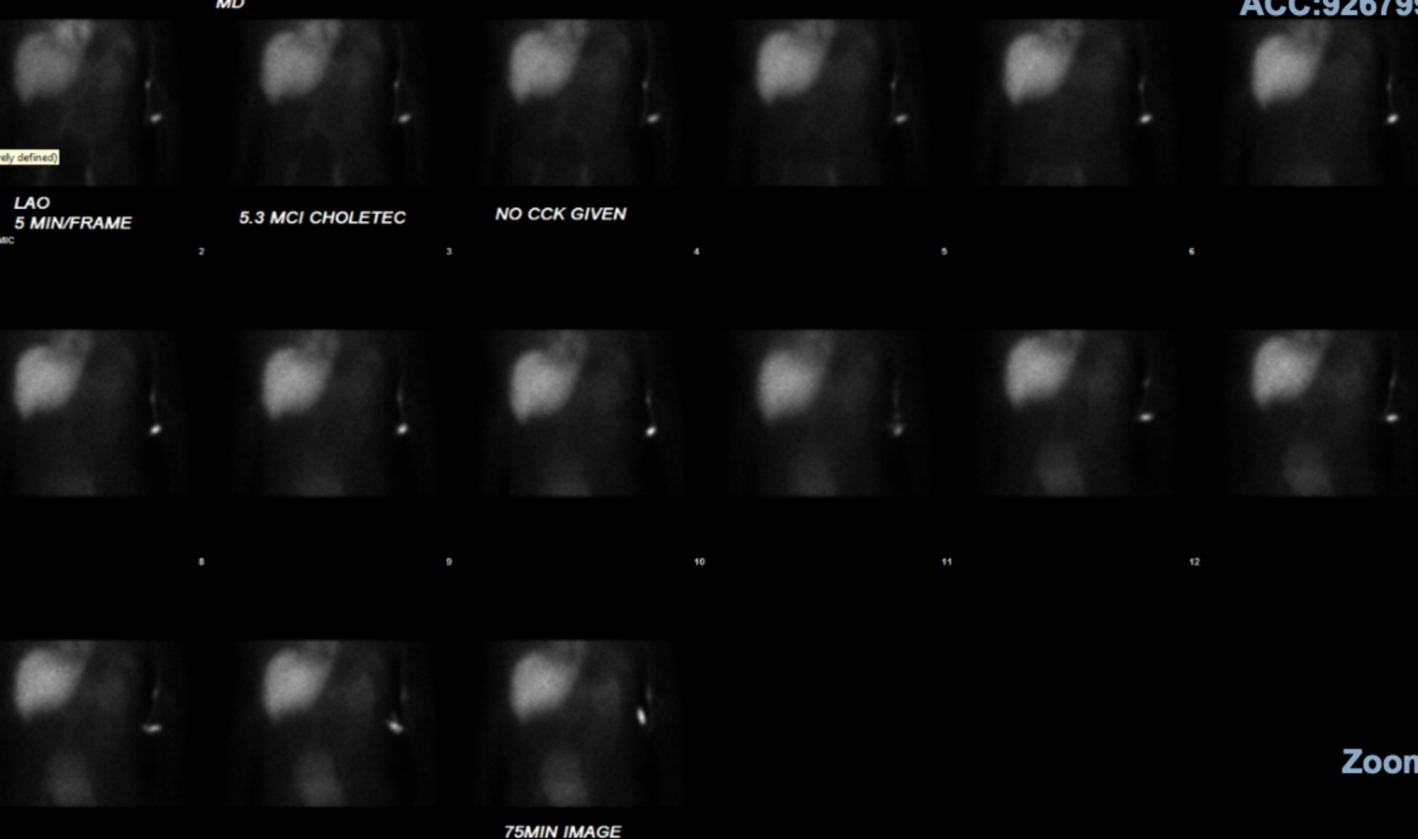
Cholescintigraphy with 99m technetium Hepatobiliary scan shows prompt hepatic uptake, prolonged hepatic activity, lack of hepatic transit, and lack of hepatic excretion—findings suggestive of primary hepatic dysfunction
